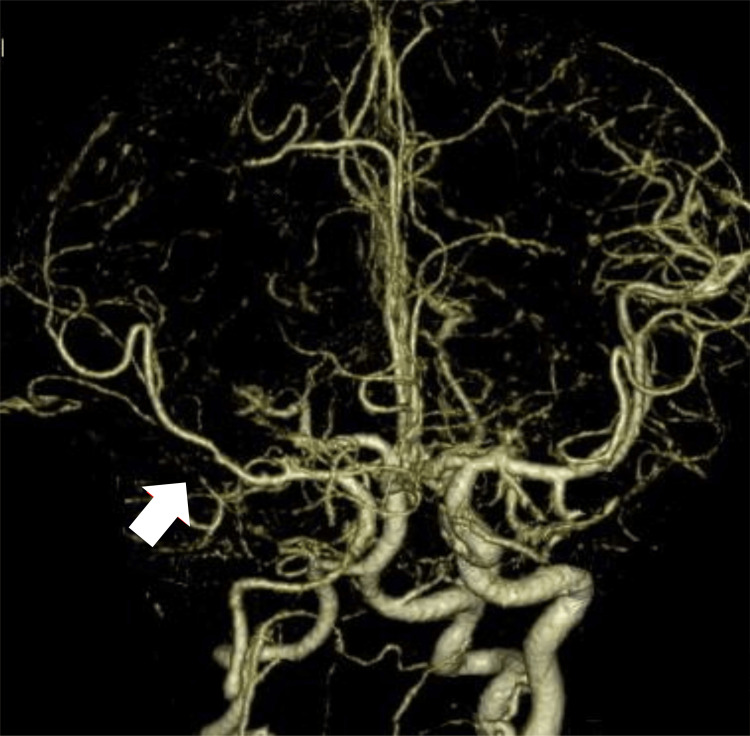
Brain three-dimensional CT angiography on day 52 (frontal view). On day 52, brain CT angiography showed improving blood flow in the peripheral segment from the M1 portion to the middle cerebral artery, with remaining stenosis over the entire length of the vessel.
